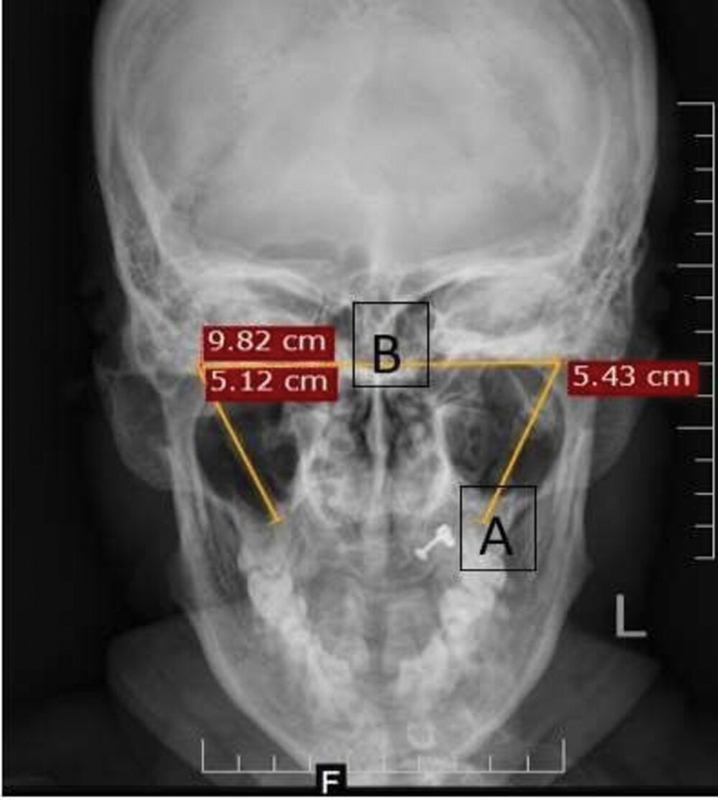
Radiograph of the styloid process (SP): (
